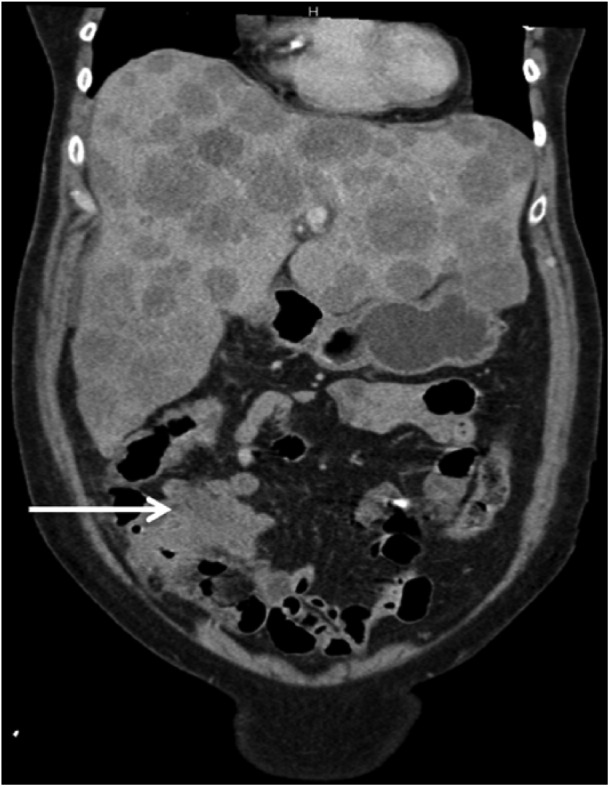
Computed tomography scan of the abdomen (coronal plane) demonstrating primary colon mass (arrow) and diffuse hepatic lesions.
